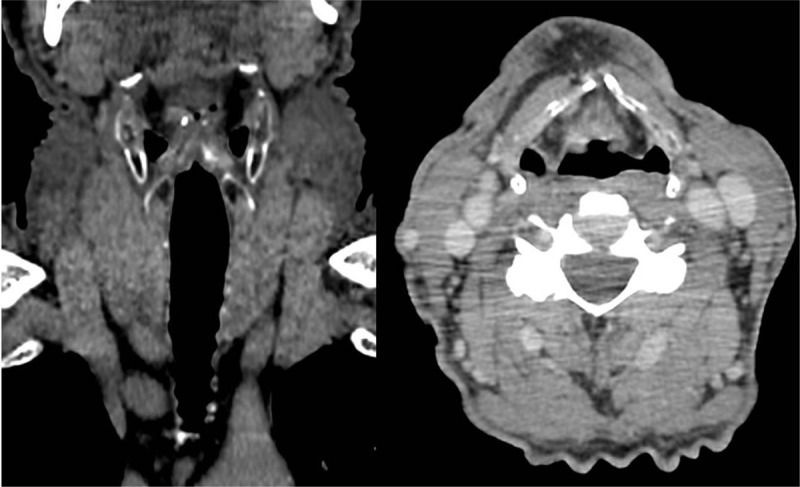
Contrast-enhanced CT scan on February 8, 2018 shows no signs of dissemination or local recurrence. CT = computed tomography.
